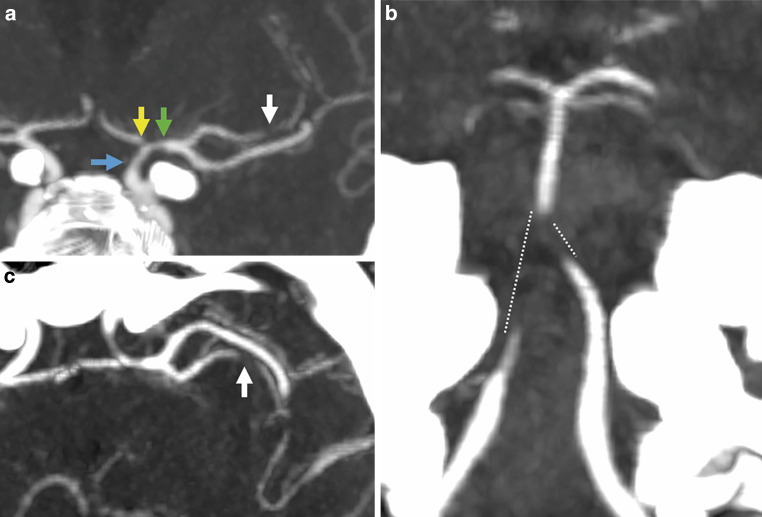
CTA of the same patient showing stenoses of the left distal ICA (a, blue arrow), the left proximal A1 segment (a, yellow arrow), the left M1 segment (a, green arrow), the left M2 segment (a, c, white arrows), both distal V4 segments, the proximal basilar artery (b, dotted lines)
